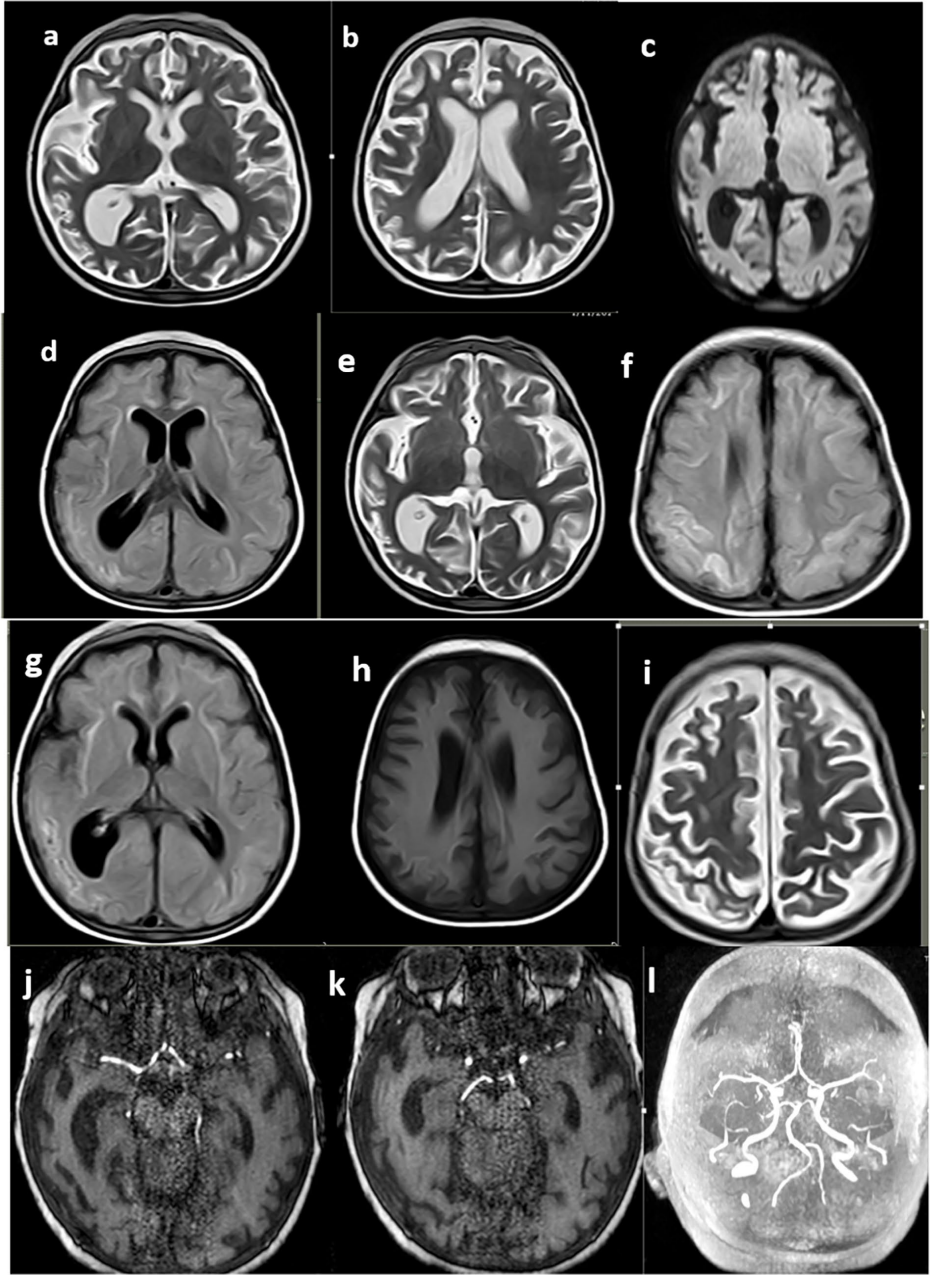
Magnetic resonance imaging manifestations in a male patient with *UNC13D* mutation, presenting with sudden disturbed conscious level and vision loss. a, b Axial T2-weighted images showing global brain atrophy corresponding with cerebral atrophy severity score of 2. c Axial diffusion-weighted image shows no definite true diffusion restriction denoting no acute vascular insult. d, e, f Axial fluid-attenuated inversion recovery (d), T2-weighted (e), and fluid-attenuated inversion recovery taken more cranially (f) images show diffuse cerebral subcortical white matter high signals. g, h, i Axial fluid-attenuated inversion recovery (g), T1-weighted (h), and T2-weighted (i) images show right occipitoparietal areas of gliosis and encephalomalacia with high T1 gyriform signal of cortical laminar necrosis of an old ischemic insult. j, k, l Axial time-of-flight magnetic resonance angiography at the anterior circulation level (j), time-of-flight magnetic resonance angiography at the posterior circulation level (k), and maximum intensity projection (l) images show diffuse irregular luminal narrowing, beading with paucity of terminal arterial arborizations, suggesting small cerebral vessel disease-like patterns
